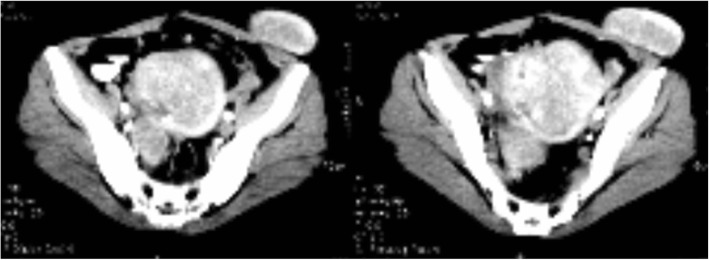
Hyperintense T2-weighted magnetic resonance imaging studies showing the parasitic left iliac fossa anterior abdominal wall myoma with cystic degeneration
